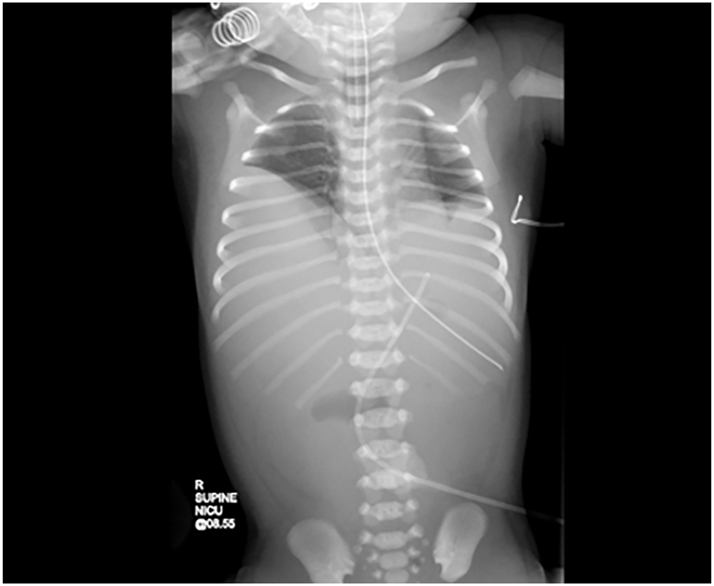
Chest radiograph demonstrating elevation of both hemidiaphragms; the small bilateral lungs are clear. There is a single dilated gas filled structure centrally in the mid-upper abdomen. The UVC is projected over the left upper quadrant of the abdomen.
